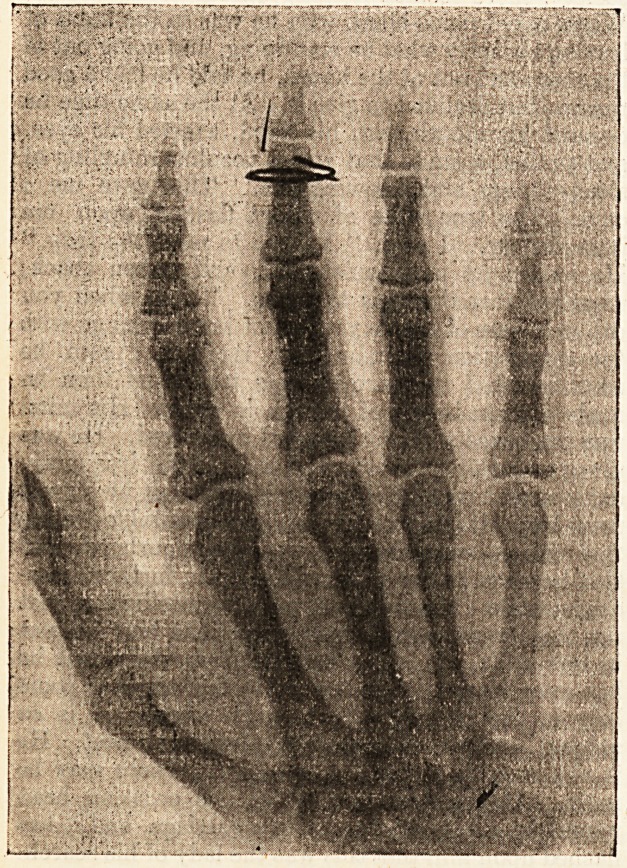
# Practical Hints for X-Ray Work
